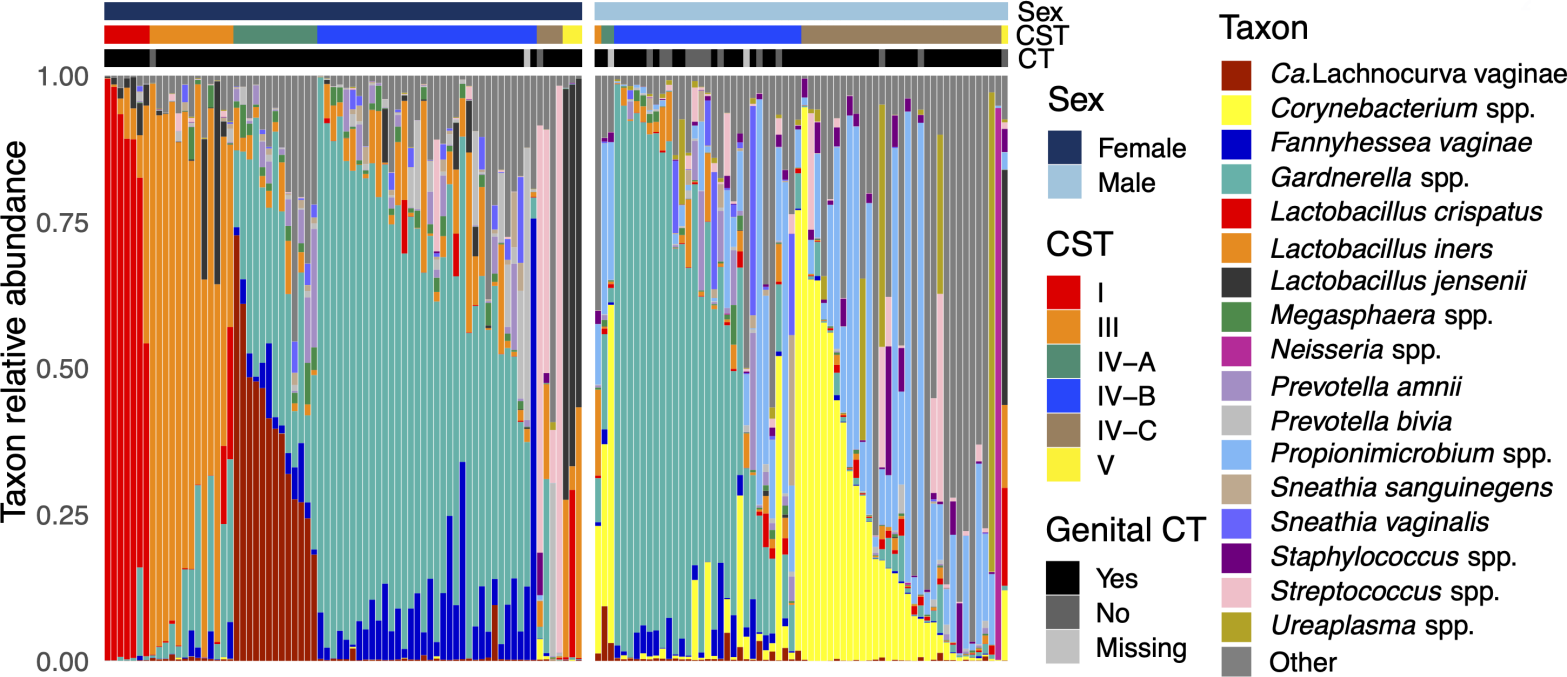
Urogenital microbiota composition, sex, and genital CT status of STING participants. Urogenital microbiota composition from whole metagenome sequencing. CSTs assigned using VALENCIA. The plot depicts the taxa with the top 10 mean relative abundances among female and male participants (calculated separately) as well as any remaining taxa that have a putative sexual transmission event.
